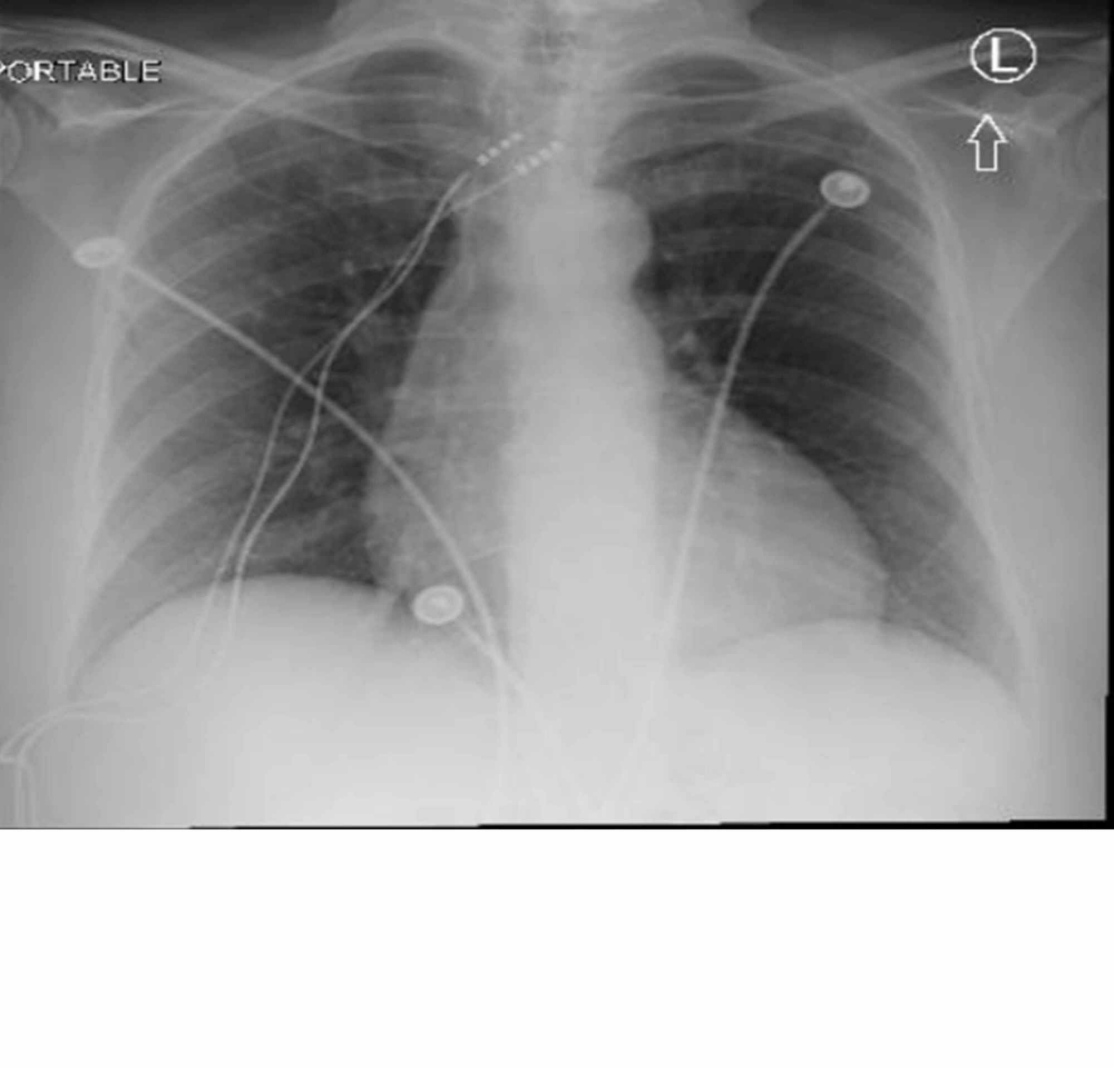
Chest X-ray Chest X-ray showing normal cardiopulmonary features.
